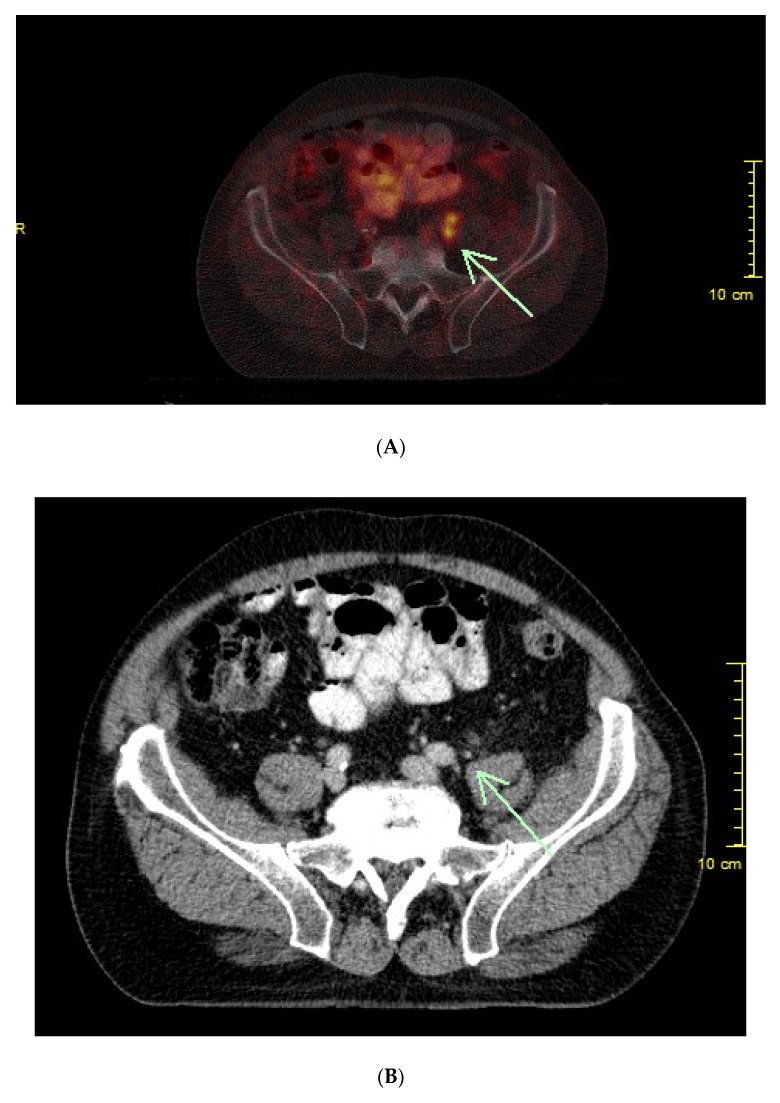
(A) History of a patient with prostate cancer followed by radical prostatectomy and lymphadenectomy (pT3, pN0, cM0, G3) in 2012. PSA doubles within three months. ^68^Ga-PSMA-11 PET/CT scan shows two lymph node metastases with high prostate specific membrane antigen (PSMA)-avidity. (B) The two small lymph node metastases are detectable on CT alone, but not suspect of malignancy.
